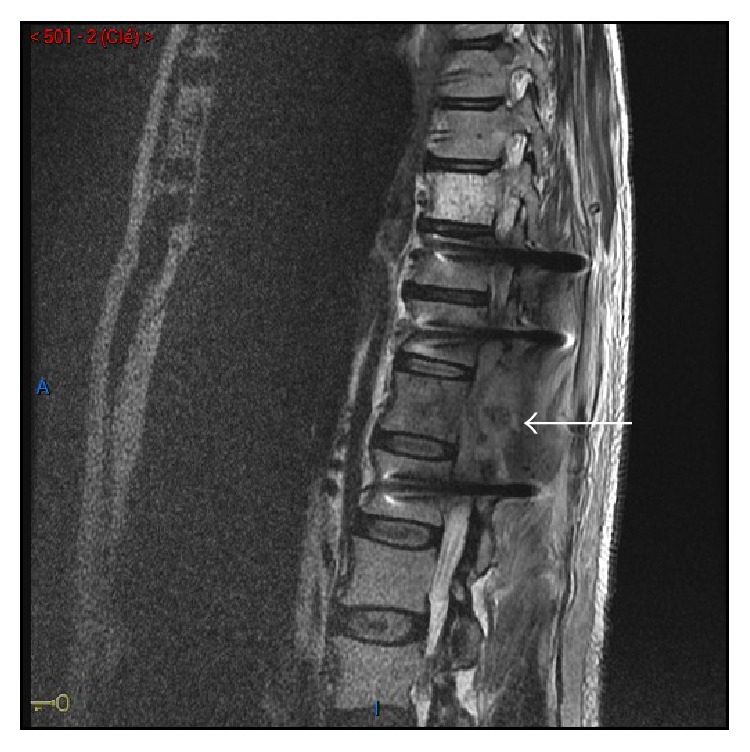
Spinal MRI revealing paravertebral lesions between T11-T12 causing significant mass effect on the conus medullaris.
